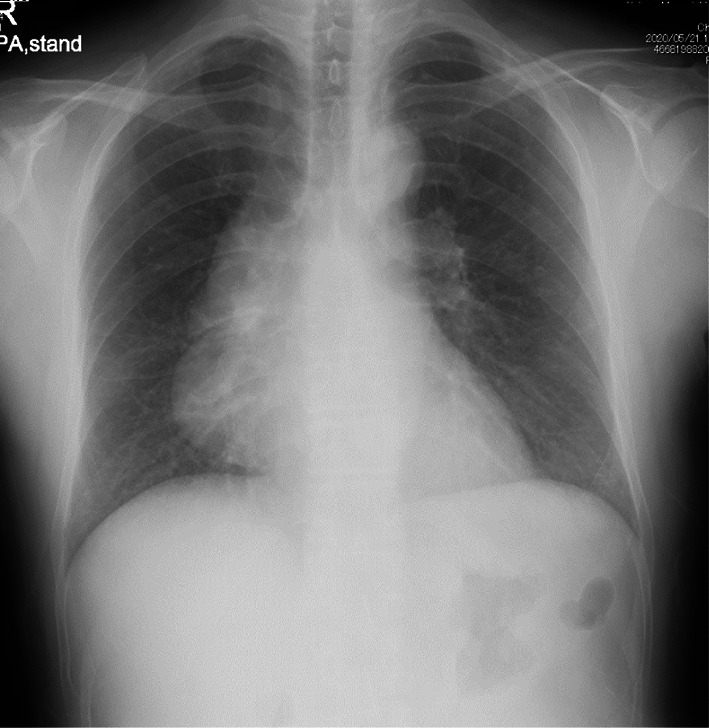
Preoperative chest X-ray showed an abnormal mass in the right pericardial region
